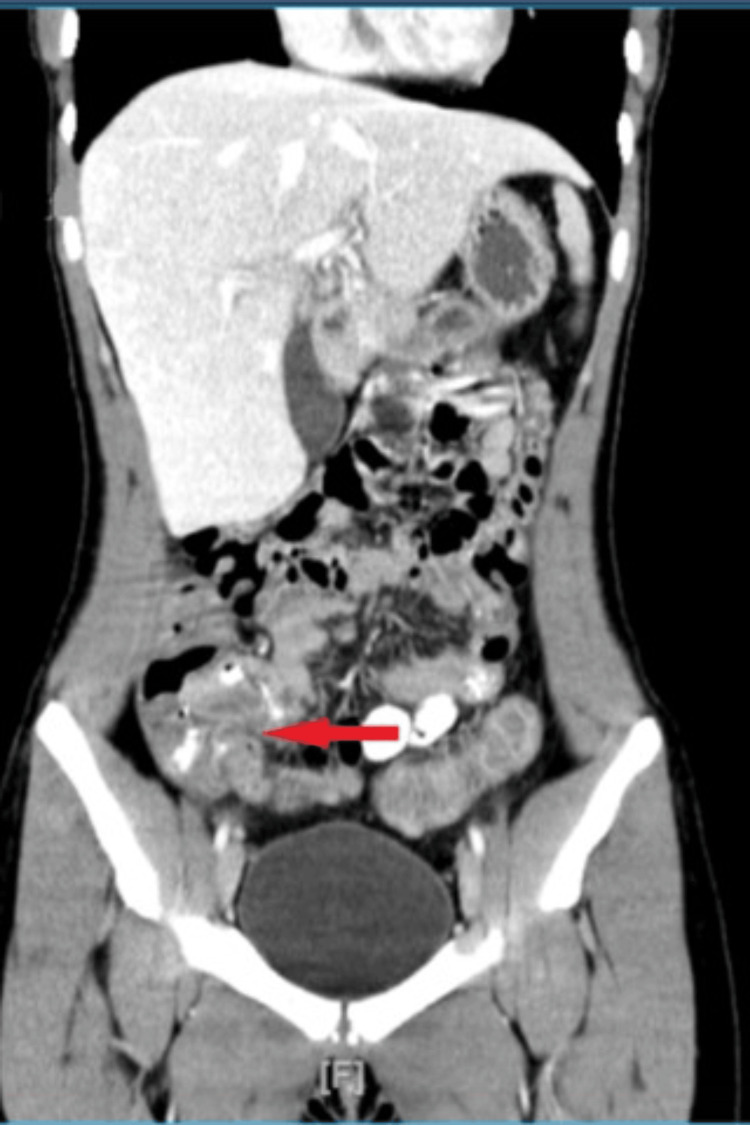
Computer tomography (CT) showing thickening of the terminal ileum (red arrow)
